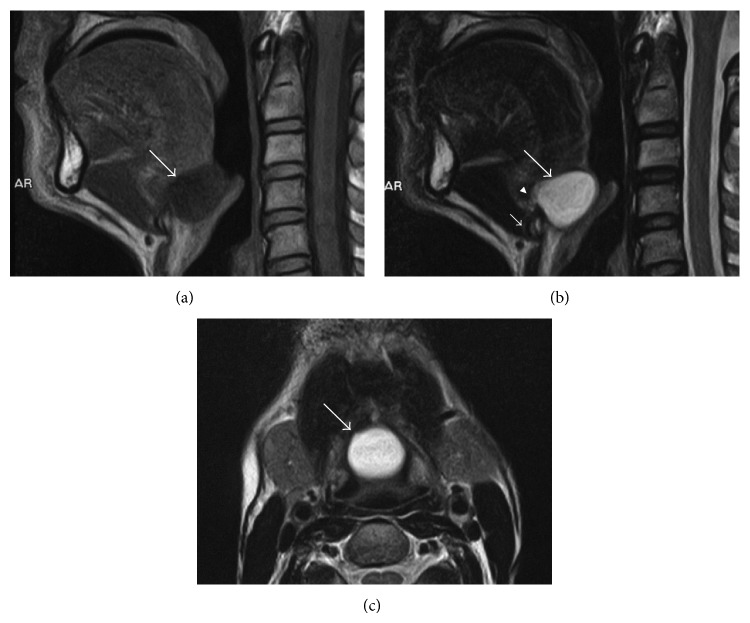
(a) Sagittal T1-weighted (TR/TE, 585/10), (b) sagittal T2-weighted (TR/TE, 4000/100), and (c) axial T2-weighted (TR/TE, 4000/100) MR images of the neck. The round mass (large arrow) has a homogenous low signal intensity in (a) and a high signal intensity in (b) and (c), suggesting a cystic lesion. In (b), there is a black and white striped tract (arrowhead) from the hyoid bone (small arrow) to the mass (large arrow), suggesting the thyroglossal duct.
